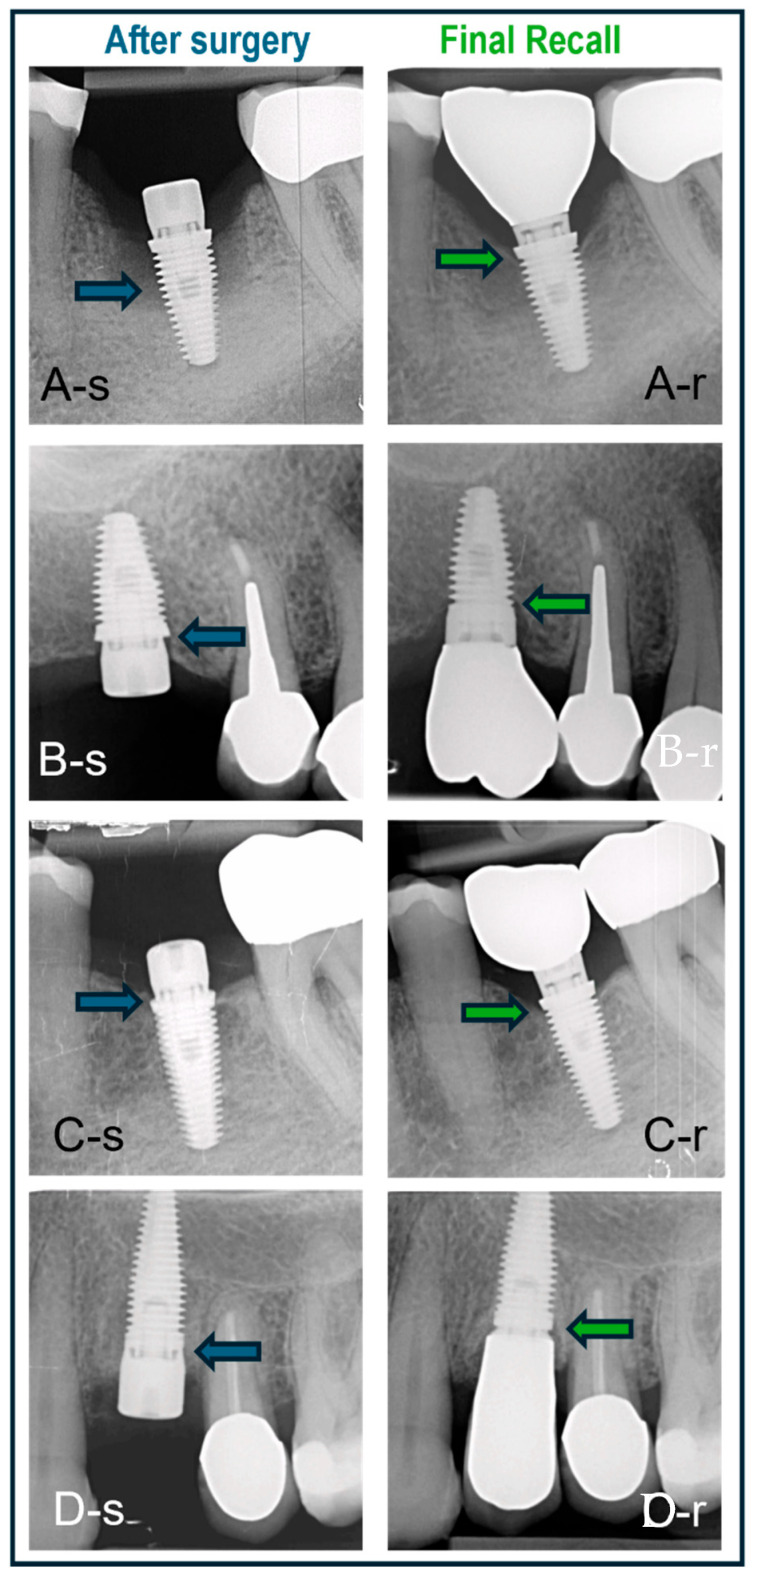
Cases treated with various surgical protocols with baseline (-s) and final recall (-r) radiographs. The blue and green arrows indicate bone level at baseline and recall, respectively. (A) Immediate placement of a lower molar using a BAT implant placed in an intact socket without guided bone regeneration; bone regrowth and bone maturation in the socket are evident. (B) BAT implant placed flaplessly in healed bone followed by minimal bone remodeling; non-platform switched crown. (C) BAT implant with open flap followed by installation of platform-switched crown and minimal bone remodeling. (D) IBT implant placed in healed bone and restored with non-platform-switched crown and no signs of bone loss.
